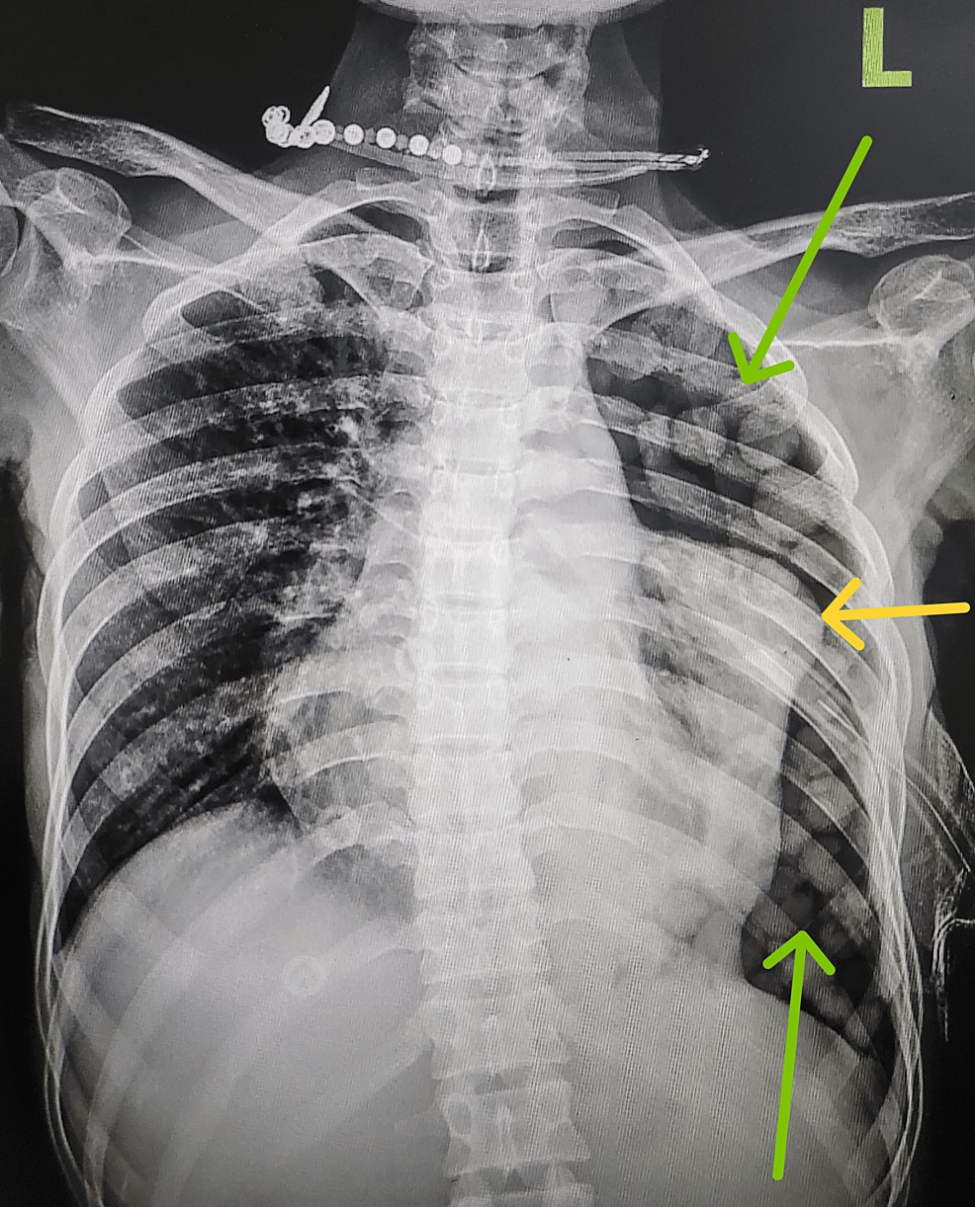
Chest X-ray suggestive of pneumothorax with multiple homogeneous rounded opacities (green arrows) and chest drain in situ in the left hemithorax. The left lung was collapsed (yellow arrow).
